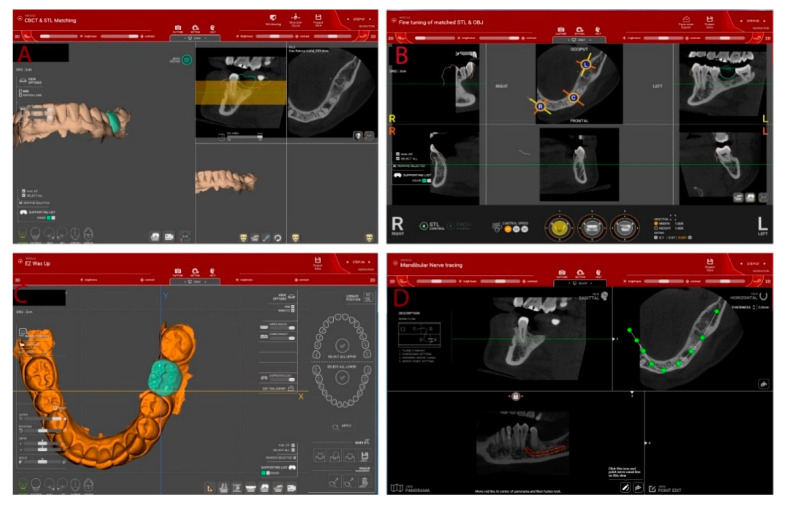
Dental implants insertion planning in R2GATE software. (A) Importing digital or digitalized impression (standard tessellation language (STL) files) and cone beam computed tomography (CBCT) Digital Imaging and Communications in Medicine (DICOM) files; (B) Superimposing files using the “best fit” algorithm; (C) Design of the prosthetic restoration (digital wax-up); (D) Marking the alveolar nerve trajectory.
